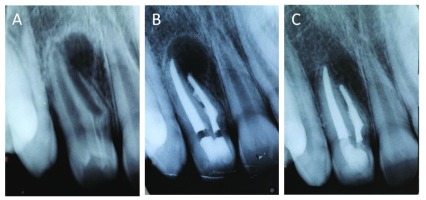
Radiographic examinations of case 1. (
